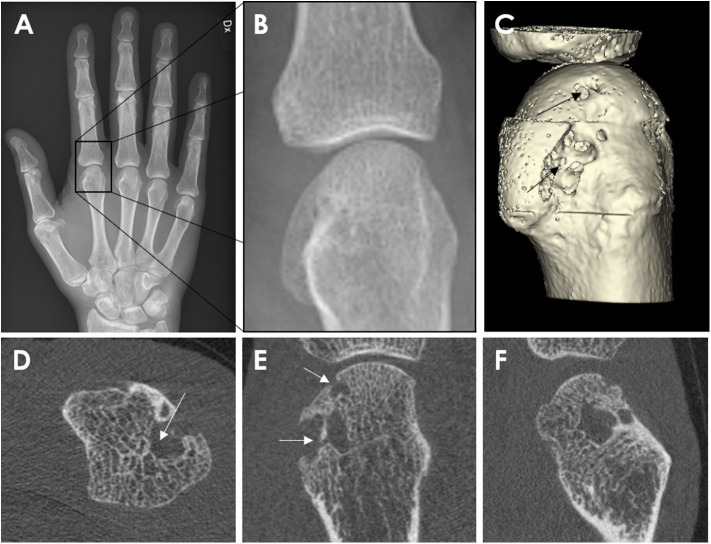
Conventional Radiography (A,B) and HR-pQCT imaging (C–E) from a 62-year-old male patient with rheumatoid arthritis. Anterior-posterior view of the right hand (A). Enlargement of the second metacarpophalangeal joint (B). High-resolution peripheral quantitative computed tomography images of the second MCP joint as 3D segmentations performed using thresholding (C) (3D Slicer, http://www.slicer.org) and the axial (D), coronal (E), and sagittal (F) plane, congruent with the corresponding radiograph. The arrow demonstrates the erosion location. Images provided courtesy of Aarhus University (RKJ, KKK, EMH).
